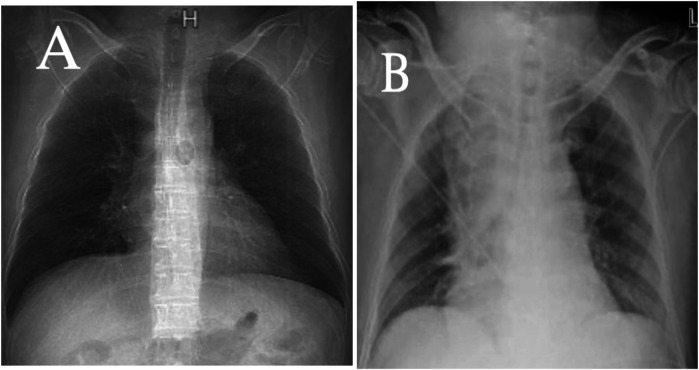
A comparison of chest x-rays taken the day before surgery (A) and on the day of surgery (B) shows a significant widening of the mediastinum in (B).
